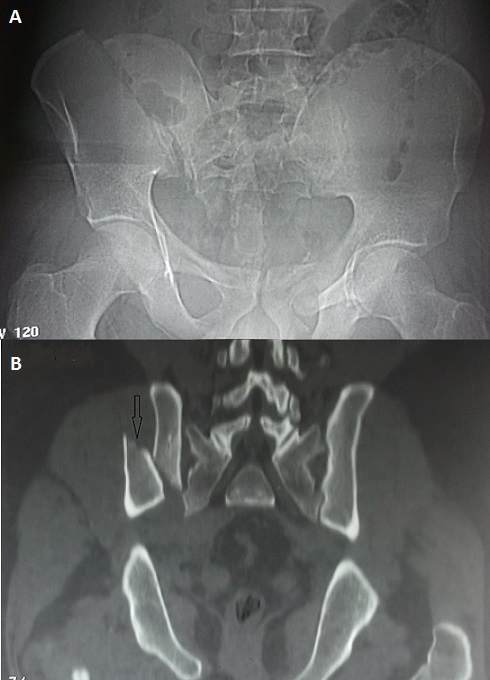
A) pelvis radio X ray showing a complete fracture of the right iliac wing; B) Pelvic CT scan showing the third fragment from the pelvic ring
